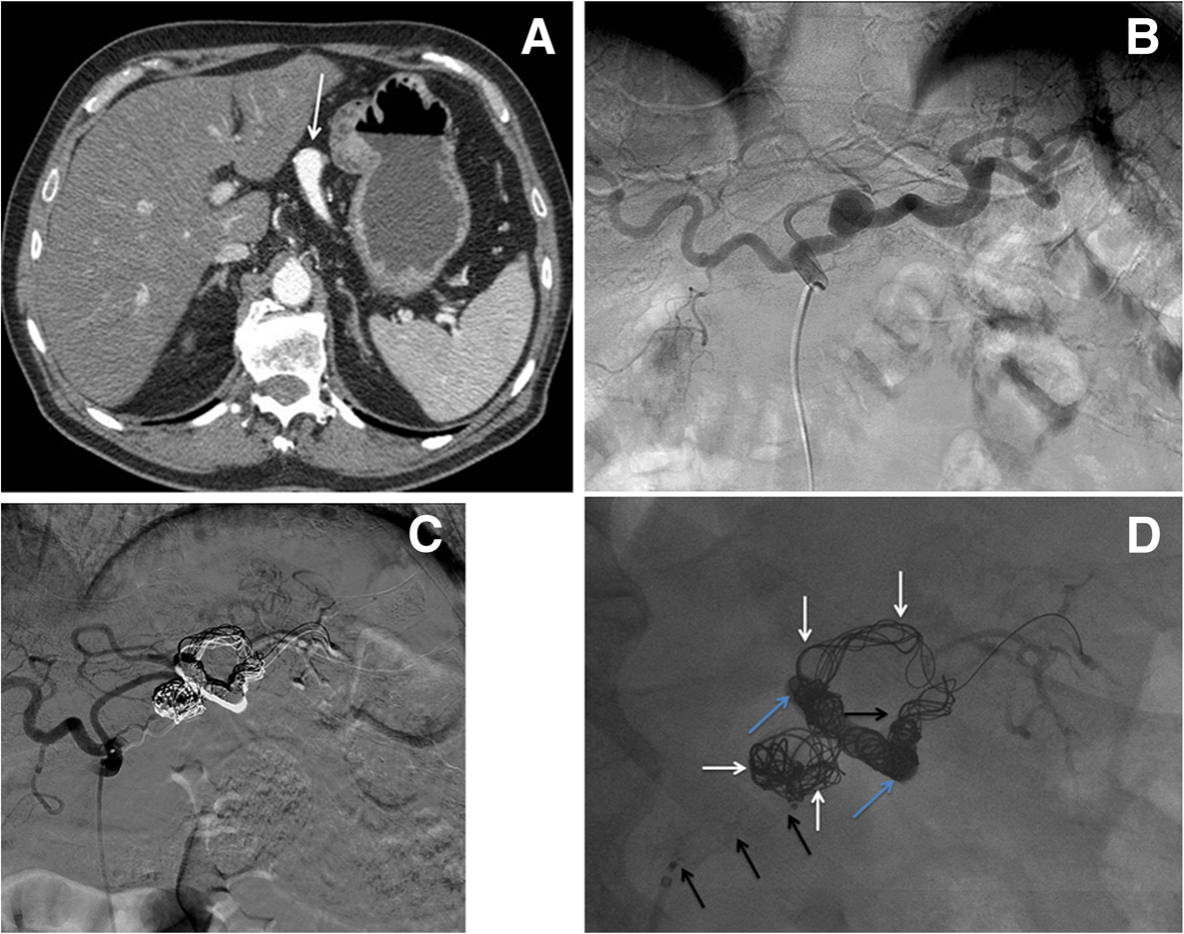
Visceral artery aneurysm (VAA) embolization. a Contrast-enhanced CT in arterial phase shows a splenic artery aneurysm (white arrow). b Transfemoral diagnostic angiography of the celiac trunk confirms the presence of the splenic artery aneurysm. c After VAA embolization, DSA shows aneurysm exclusion, splenic artery occlusion with preserved patency of its distal branches through gastric collaterals. d X-rays show embolic agents used for splenic artery aneurysm embolization: Squid (light blue arrows), coils (white arrows) and Amplatzer plug (black arrows)
